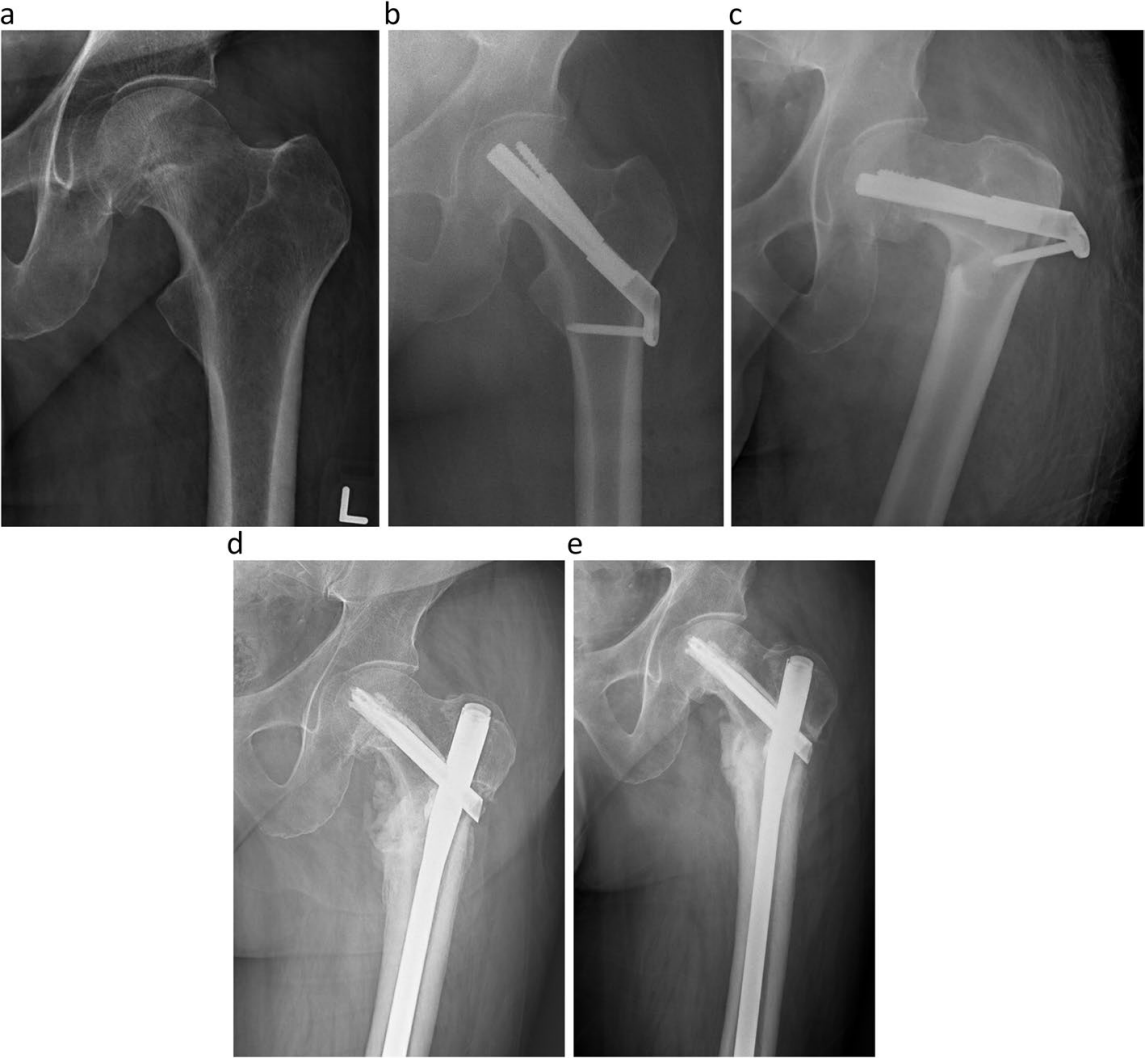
Panel a is an anteroposterior radiograph of the left hip showing an incomplete nondisplaced fracture of the femoral neck on the tension side of the femoral neck. Panel b is an anteroposterior radiograph of the hip after successful implantation of FNS. Panel c is an anteroposterior radiograph of the left hip showing a peri-implant subtrochanteric fracture at the level of the distal screw. Panel d is an anteroposterior radiograph of the left hip taken 9 months after implantation of cephalomedullary nail with painful nonunion. Panel e is a radiograph taken 1 year postoperatively after removal of distal interlocking screws showing callus formation
